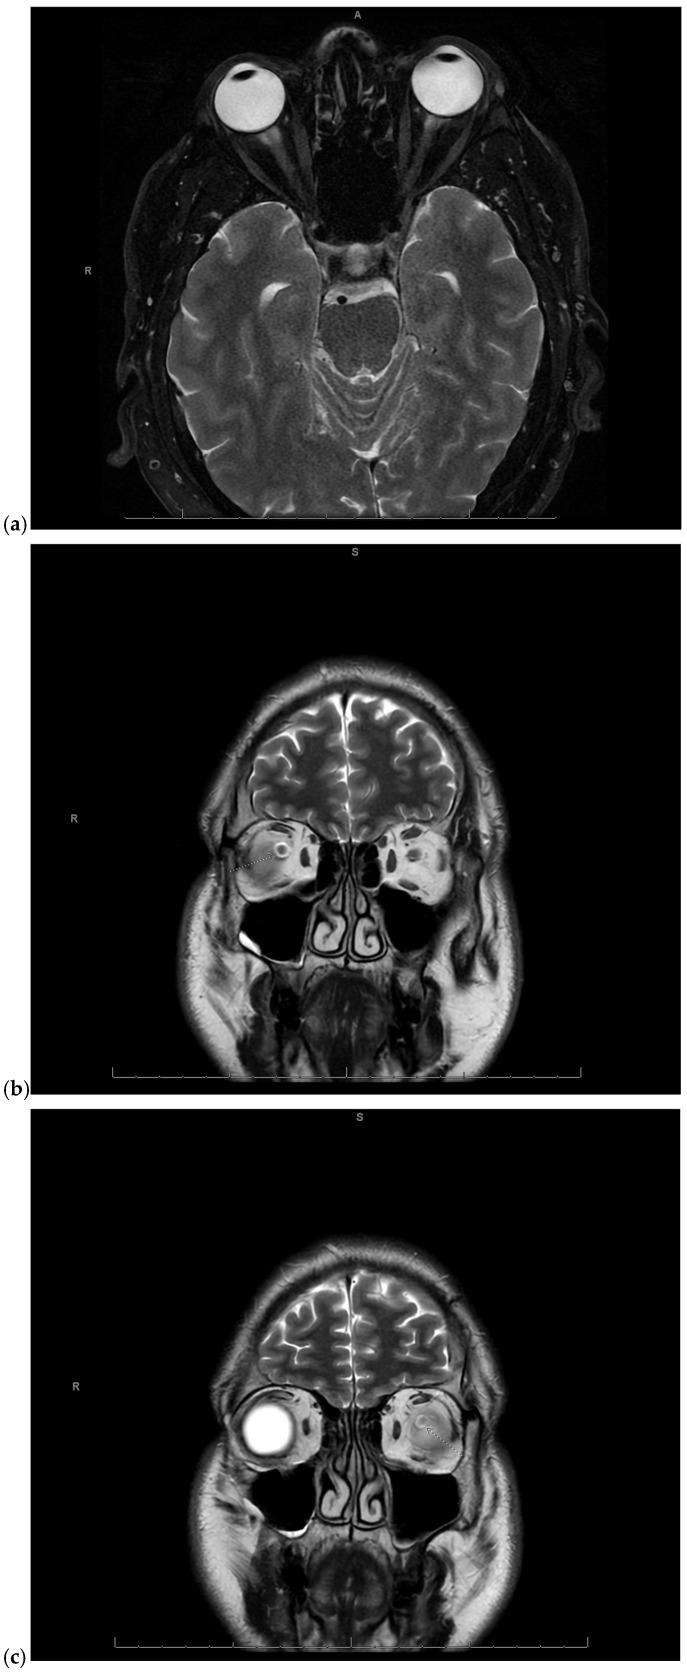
(a) Magnetic Resonance Imaging (MRI) of the brain and orbits fat-saturated T2-weighted axial image showing mild right greater than left T2 hyperintense signal in the periphery of the optic nerves in the anterior intraorbital region, consistent with optic neuritis. A: anterior, R: right; (b) MRI of the brain and orbits coronal T2-weighted image showing right sided optic neuritis. S: superior, R: right; (c) MRI of the brain and orbits coronal T2-weighted image showing left sided optic neuritis. S: superior, R: right.
